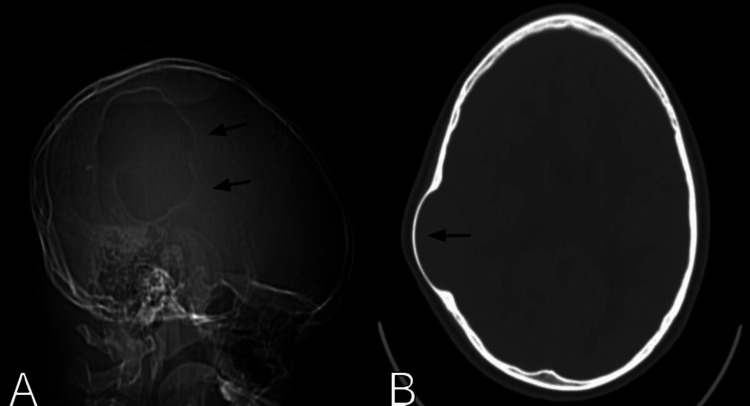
Head CT scan skull scout view (A) and bone window axial view (B) showing the classic bone thinning and scalloping (black arrows).
